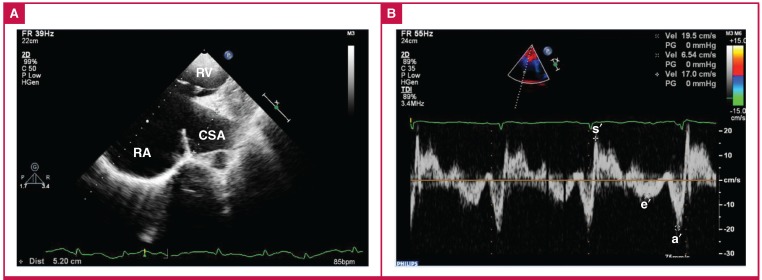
Transthoracic echocardiography performed four years prior to presentation showed: A. the aneurysmal dilated CS was 5 cm in diameter; B. tissue Doppler imaging velocities at the septal mitral annulus: early diastolic myocardial velocity (e′) was 6.54 cm/s and systolic myocardial velocity (s′) was 19.5 cm/s. RV, right ventricle; RA, right atrium; CSA, coronary sinus aneurysm; a′, late diastolic myocardial velocity.
